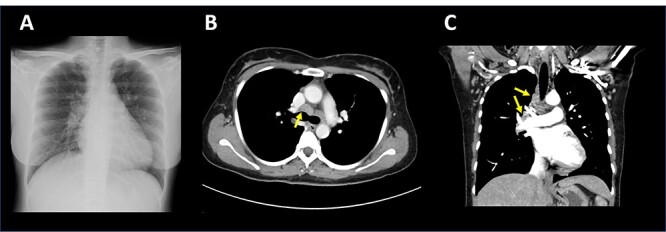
Chest X-ray on admission (A) and chest computed tomography on Day 7 (B, C). Enlarged lymph nodes indicated with yellow arrows.
